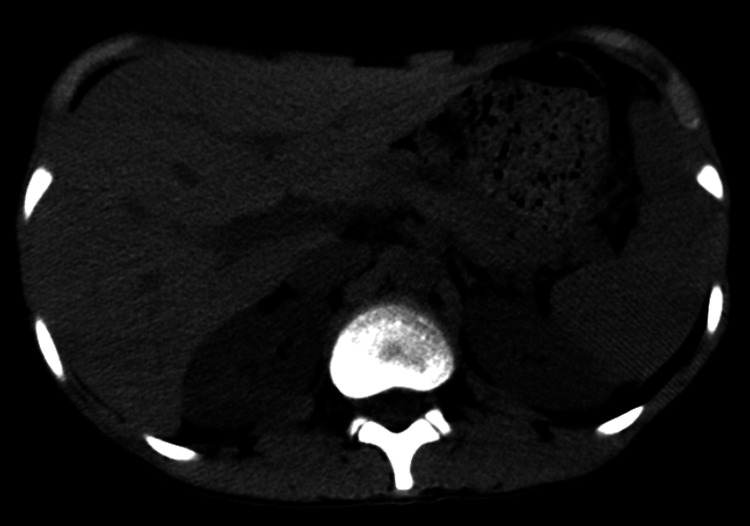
Baseline noncontrast CT chest axial images. Partially covered liver shows no lesions. It should be noted that a noncontrast CT is suboptimal for detecting hepatic metastases
